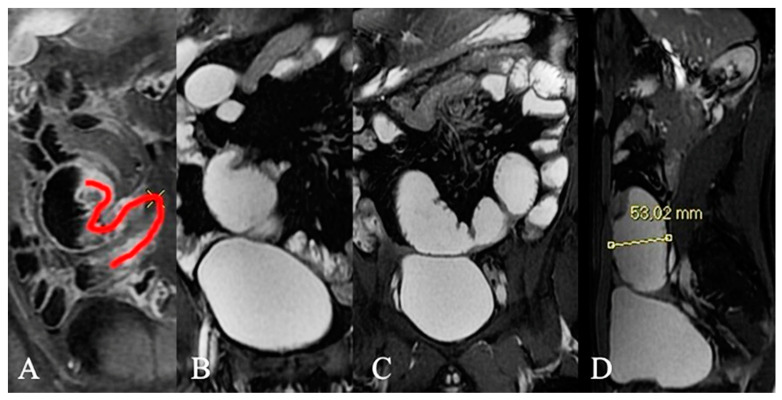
Very functionally significant stenosis: upstream bowel dilatation greater than 3 cm with constant and permanent ectasia of upstream bowel loops before high-grade bowel stenosis. Contrast-enhanced fat-suppressed T1-weighted image (A): The freehand red curve represents a relatively long, markedly stenotic segment of the ileum with marked enhancement, rigid and non-expandable. Permanent ectasia of upstream bowel loops before high-grade bowel stenosis in coronal (B,C) and sagittal (D) MRE in the same patient.
